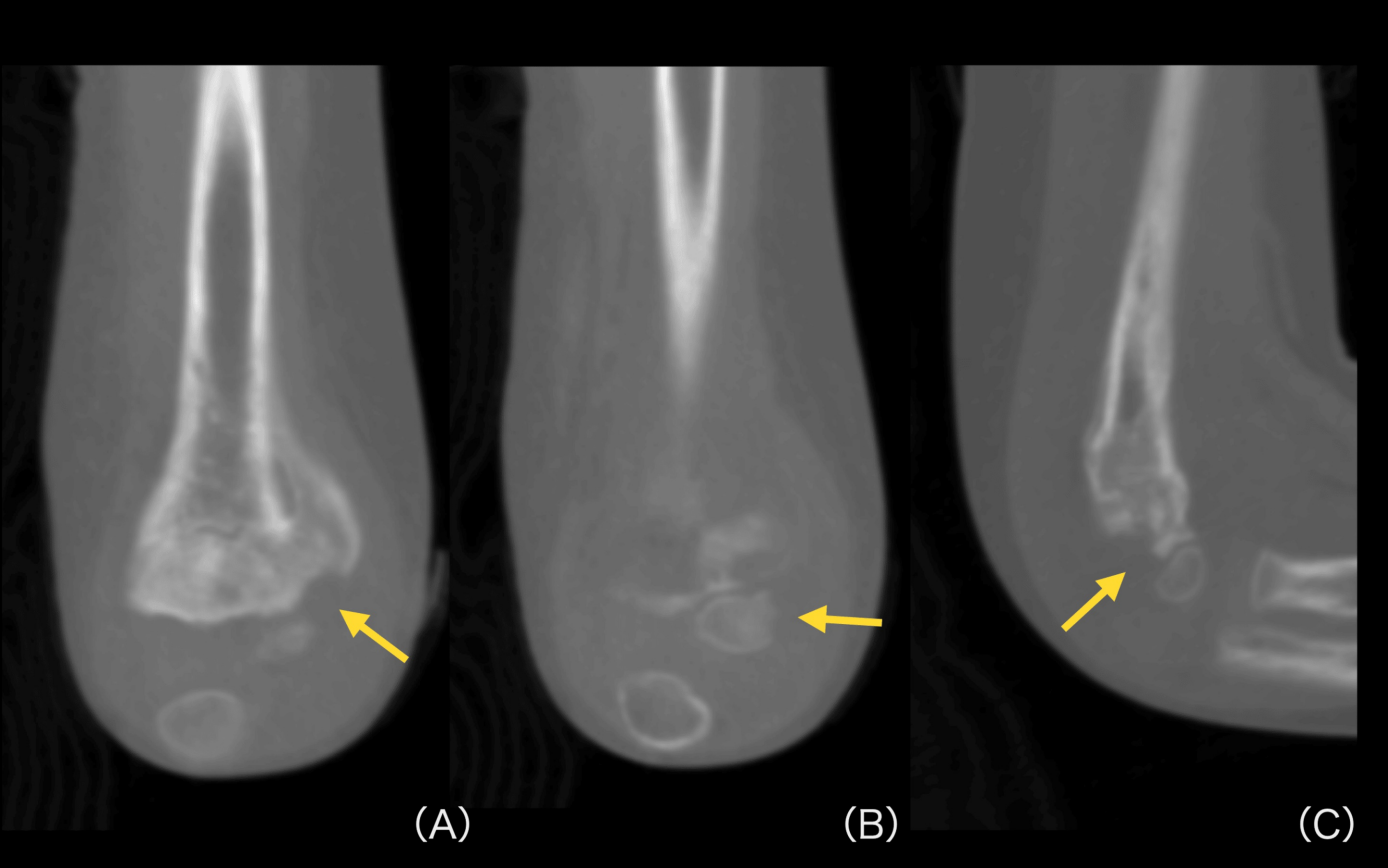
Coronal and sagittal CT demonstrating cortical breach and bone defect Coronal and sagittal CT images of the right elbow demonstrating a cortical bone defect in the posterolateral distal humerus: coronal view at the level of the defect (A), coronal view at an adjacent level (B), and sagittal view (C). Yellow arrows indicate the bone defect; the loss of cortical continuity confirms infective bone destruction rather than malreduction of the fracture or unossified cartilage. These findings correspond to the cavitary lesion visualized on three-dimensional reconstruction (Figure 5).
